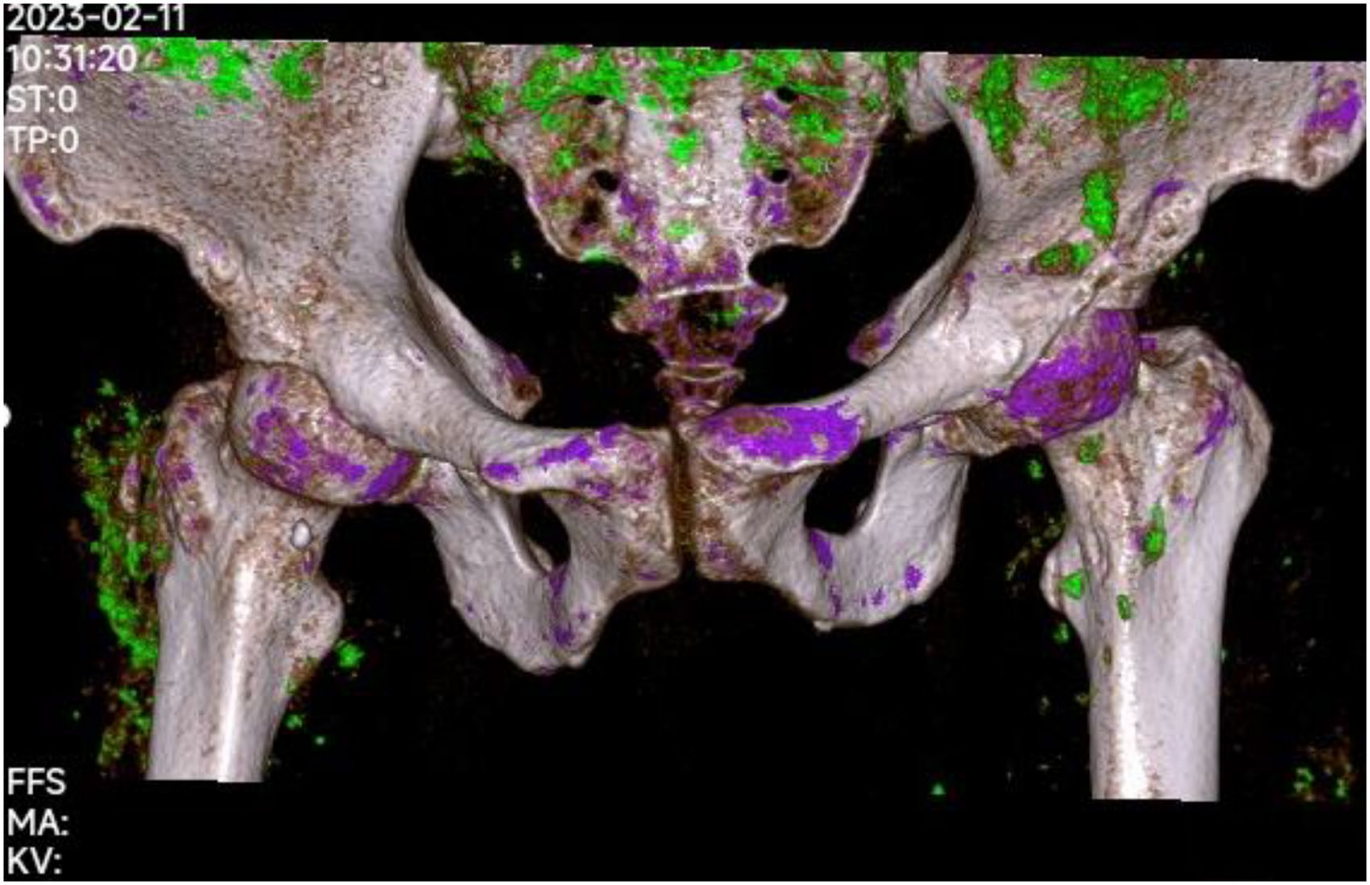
Dual-energy CT scan of pelvic 9 days after admission showed multiple gout nodules around bilateral iliac bone and sacrum and the proximal femur.
